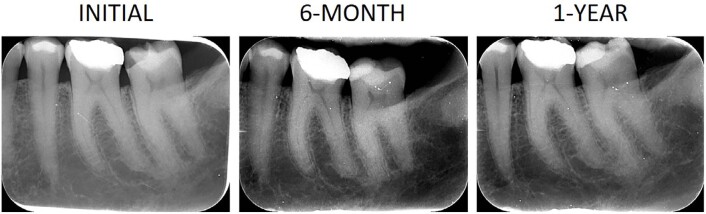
Representative radiographic follow-up of the study, performed at the initial time point (pre-restoration), 6 months, and 1 year after the restoration.
